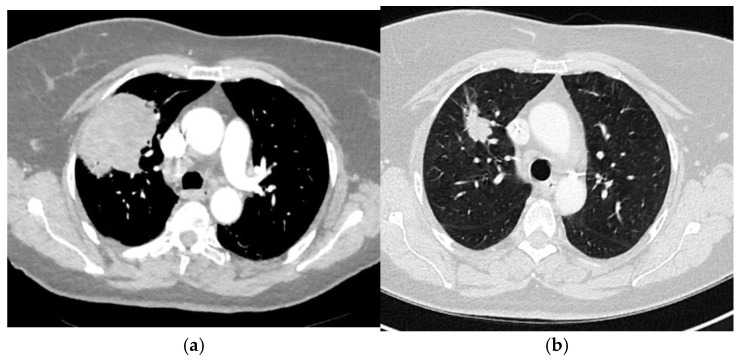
Axial computed tomography images showing an isolated pulmonary relapse: (a) before induction chemotherapy; (b) after induction chemotherapy.
